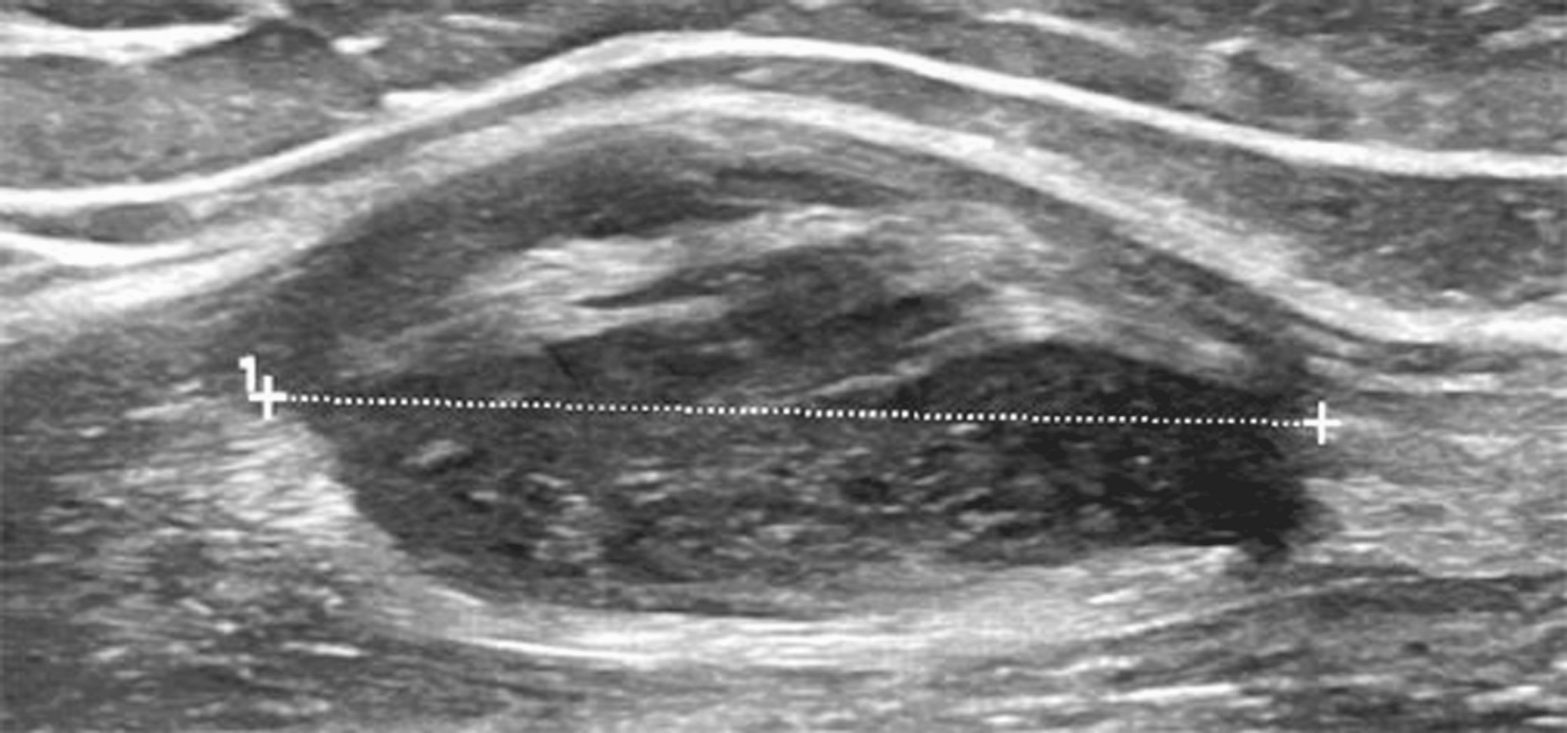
Ultrasound of palpable mass in the left arm shows spindle-shaped intramuscular lesion measuring 27 mm.
